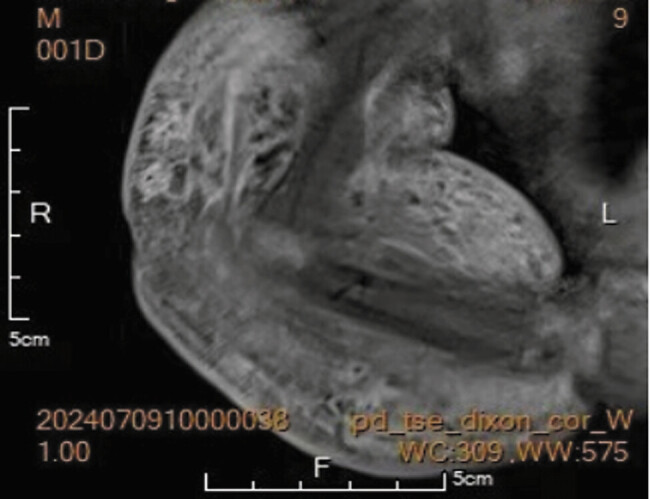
Magnetic resonance imaging findings of vascular tumor infiltration with hemorrhage.
